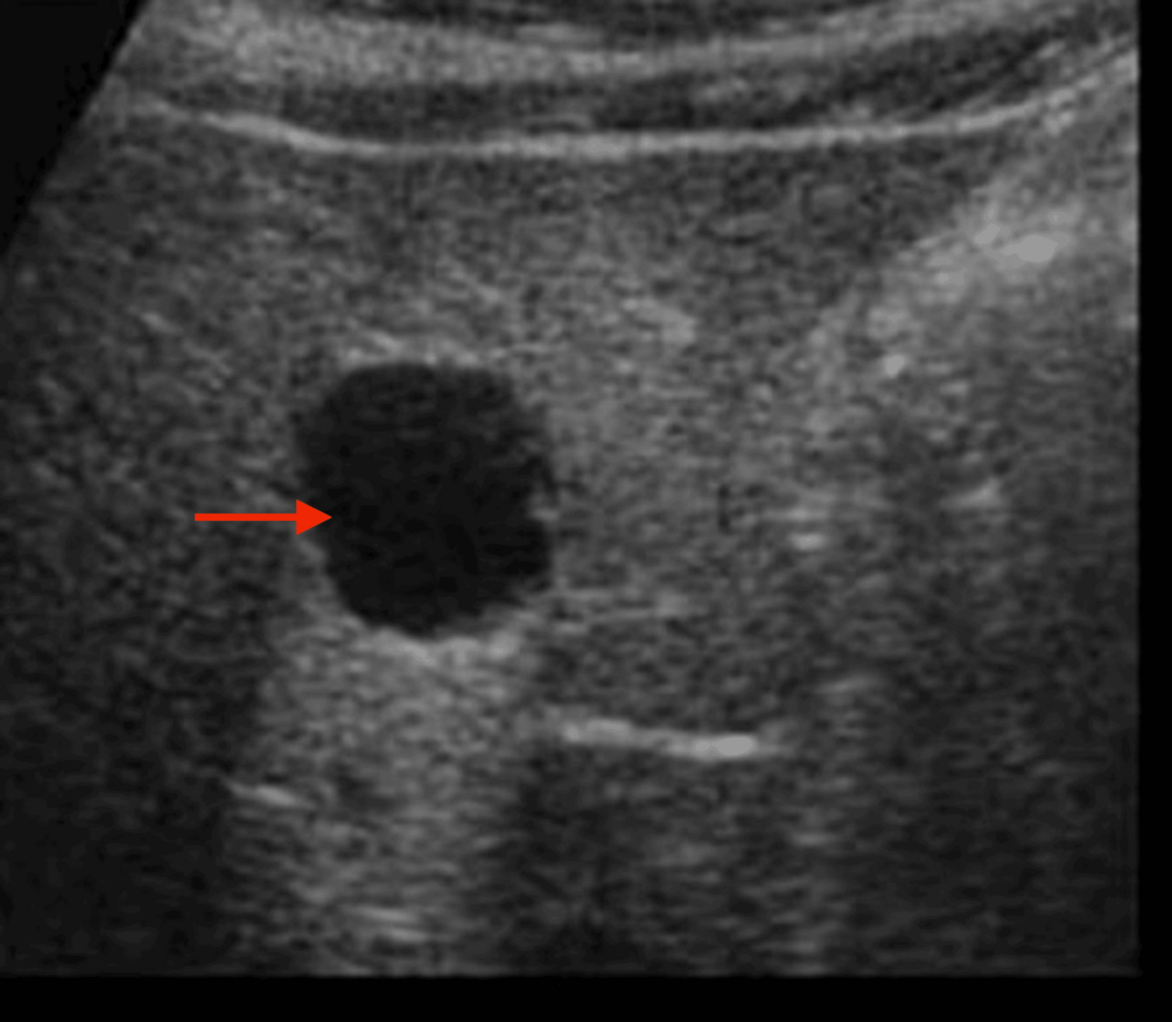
Ultrasound of the liver Transverse abdominal ultrasound showing a well-circumscribed anechoic cystic lesion within the hepatic parenchyma, consistent with a benign simple hepatic cyst (red arrow)
